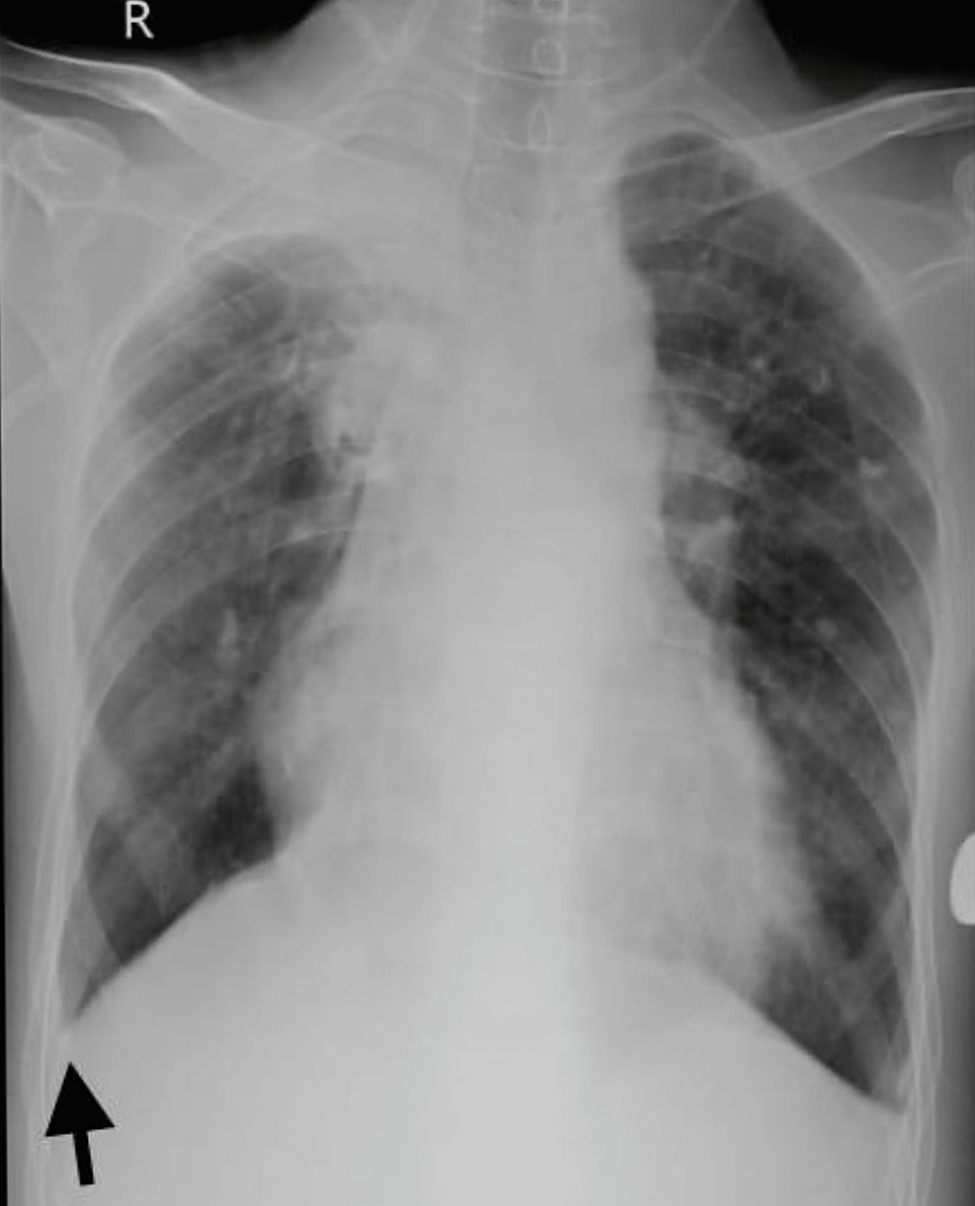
Chest X-ray in postero-anterior view; the arrow shows blunting of right costophrenic angle, which is suggestive of pleural effusion
